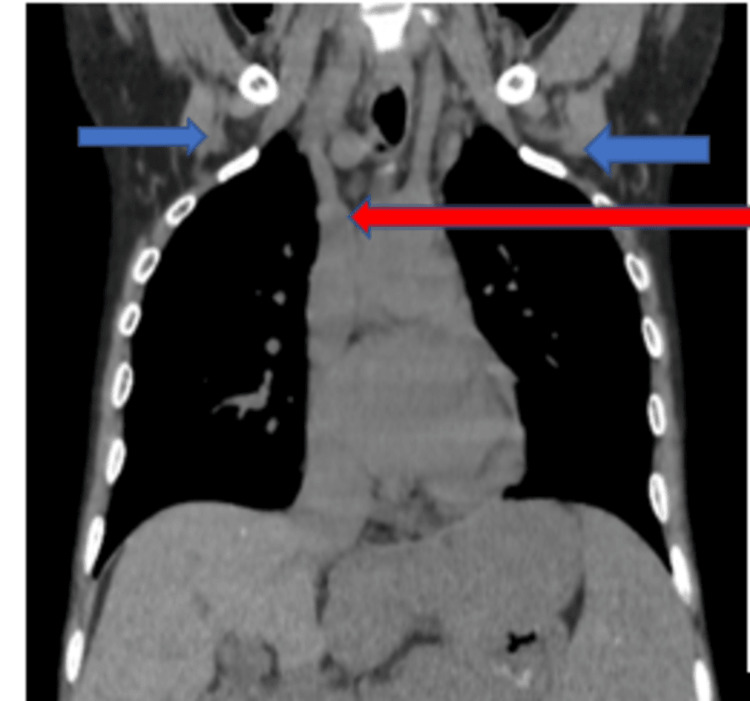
High-resolution computed tomography (HRCT) of the thorax showing healing of the tracheal fistula (red arrow) with complete resolution of the subcutaneous emphysema (blue arrow)
